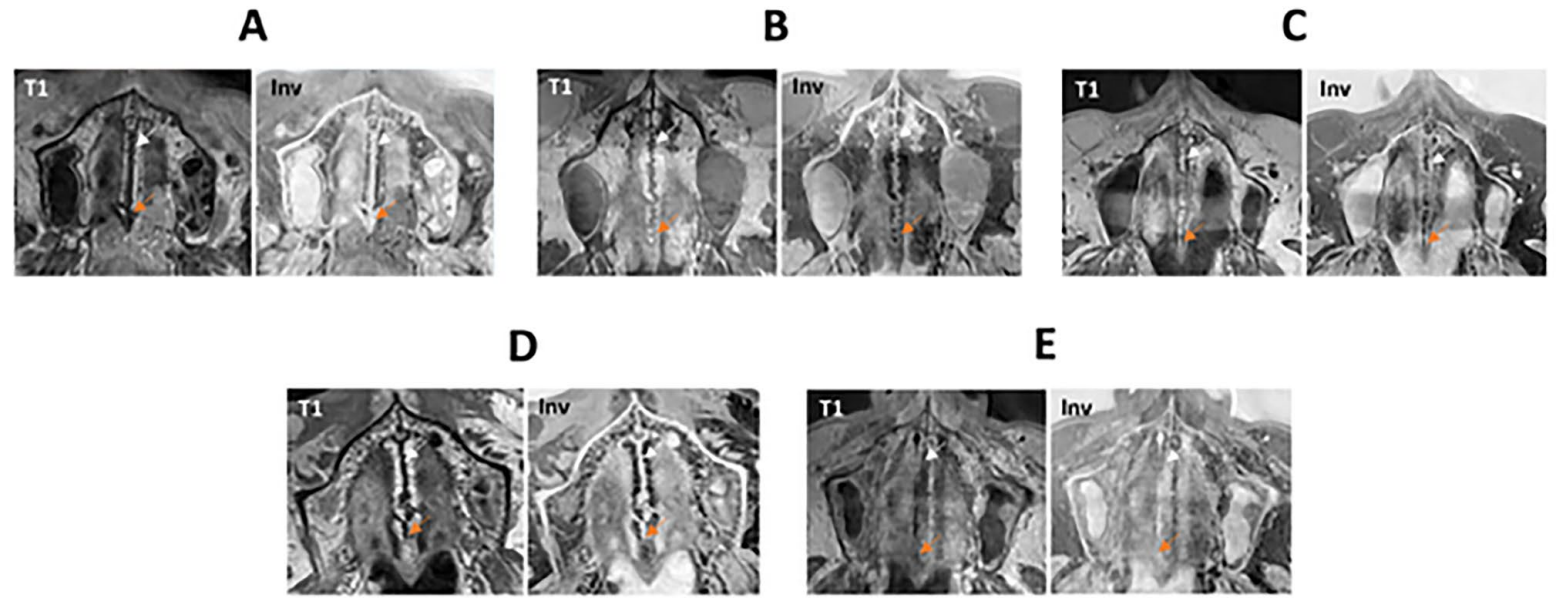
Displays the 5 different maturation stages A–E as obtained on 3 T MRI (T1 = original T1w 3 T MRI; B: inverted 3T MR image). In stages A–C, the suture is visible in both the maxillary (white arrow in A–E) and palatine bones [17](yellow arrow in A–E). A Stage A: the suture is straight high-density line with no or little interdigitation [17]. B Stage B: the suture is depicted as a scalloped high-density line with an irregular shape [17]. C Stage C: the suture comprised two parallel, scalloped, high-density lines close to each other [17]. D Stage D: sutural fusion has commenced in the palatine bone [17] (yellow arrow). E Stage E: the suture is totally fused and is neither visible in the maxillary (white arrow) nor palatine bones (yellow arrow) [17]
